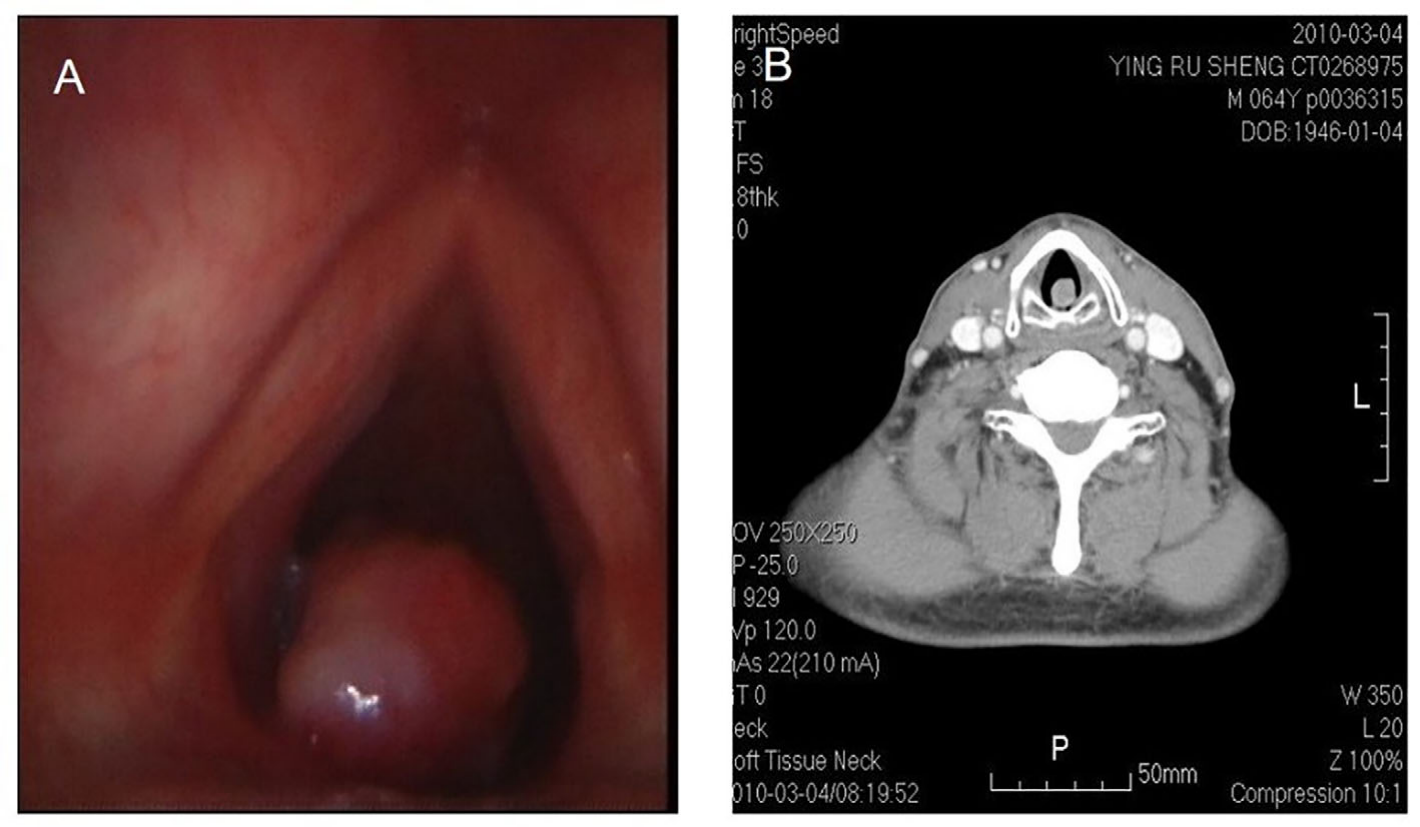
Fibrosarcoma in throat. Laryngoscope shows a nodulated neoplasm in the left posterior wall of hypolarynx, with surface relatively flattening (A). CT also shows that CT a nodulated neoplasm in infraglottic portion, with medium isotropical enhancement after contrast. The lesion is connected with posterior wall (B).
